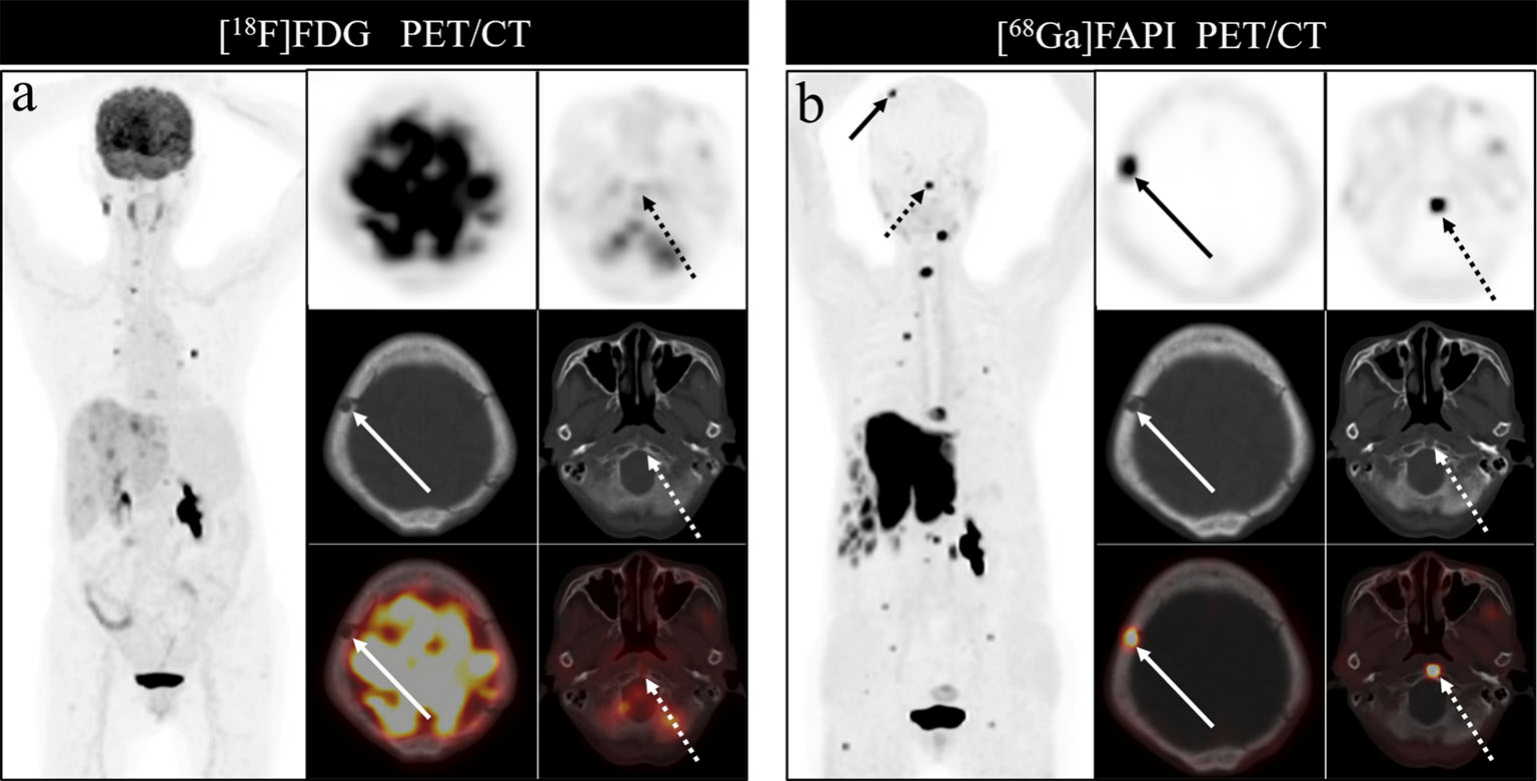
A 76-year-old woman underwent [^18^F]FDG and [^68^Ga]Ga-DOTA-FAPI-04 PET/CT (A, B) to assess possible recurrence of thyroid cancer. The MIP image (A, B) of [^18^F]FDG and [^68^Ga]Ga-DOTA-FAPI-04 PET/CT demonstrated multiple tracer activities bone and liver lesions. On the selected axial images, [^18^F]FDG PET/CT (A) showed limited [^18^F]FDG uptakes in the parietal bone (arrows) and clivus (dashed arrows), while [^68^Ga]Ga-DOTA-FAPI-04 PET/CT (B) showed intense [^68^Ga]Ga-DOTA-FAPI-04 uptakes in the parietal bone (arrows) and clivus (dashed arrows). In addition, the expression of [^68^Ga]Ga-DOTA-FAPI-04 in liver metastasis was significantly higher than that of [^18^F]FDG.
